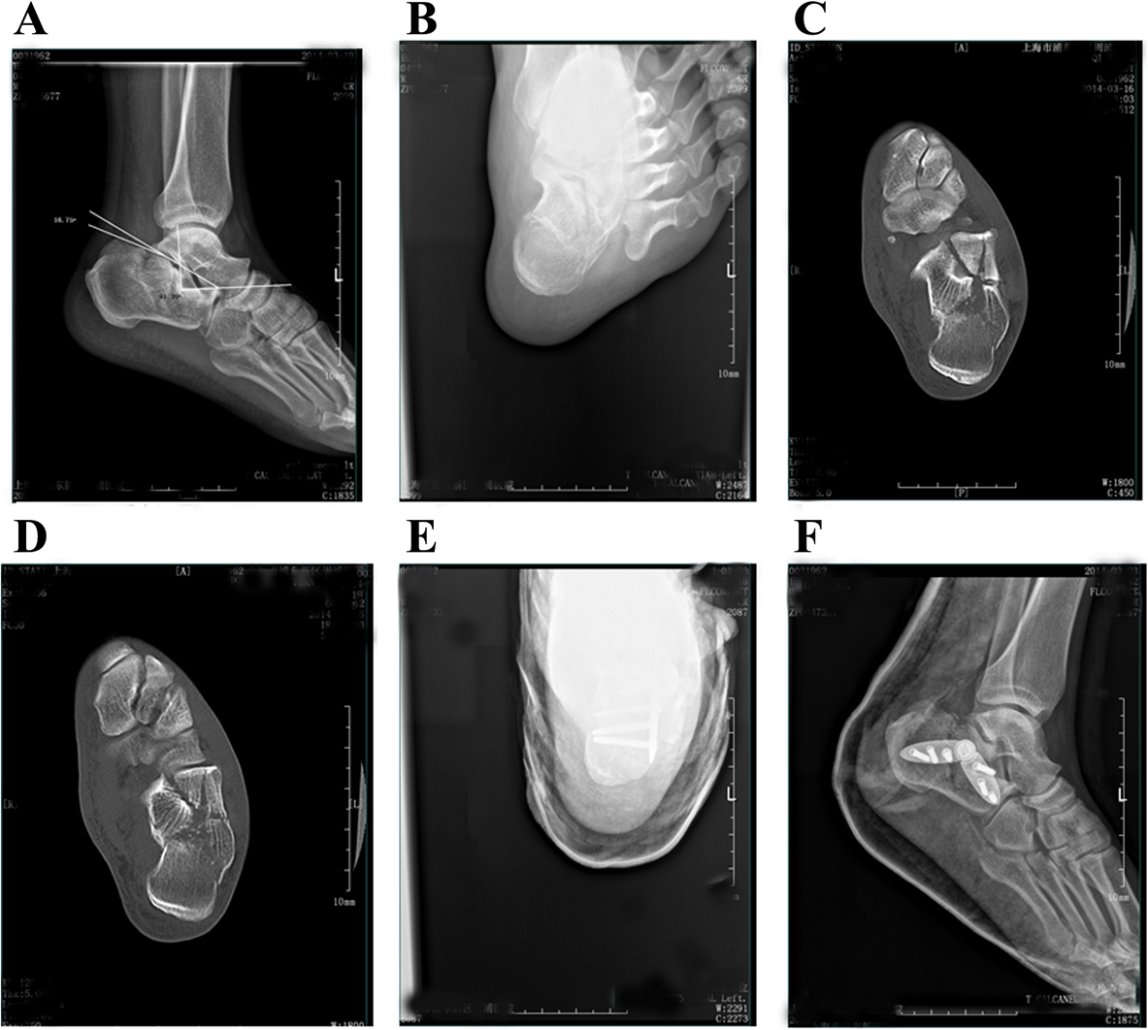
A 52-year-old male patient was admitted to our hospital because of pain and swelling on the right foot after falling from a height. Preoperative lateral (a) and axial (b) radiographs showing the right calcaneal fracture, with reduced Böhler angle (16.75°), Gissane angle (91.39°), calcaneal length (58.23 mm), and calcaneal height (38.59 mm) but increased calcaneal width (38.59 mm). Preoperative horizontal (c) and coronal (d) computed tomography images showing a Sanders IIC fracture. Postoperative lateral (e) and axial (f) radiographs showing obvious corrections of the Böhler angle (23.17°) and Gissane angle (124.4°) and improvements in calcaneal length (70.28 mm), height (35.6 mm), and width (35.54 mm)
